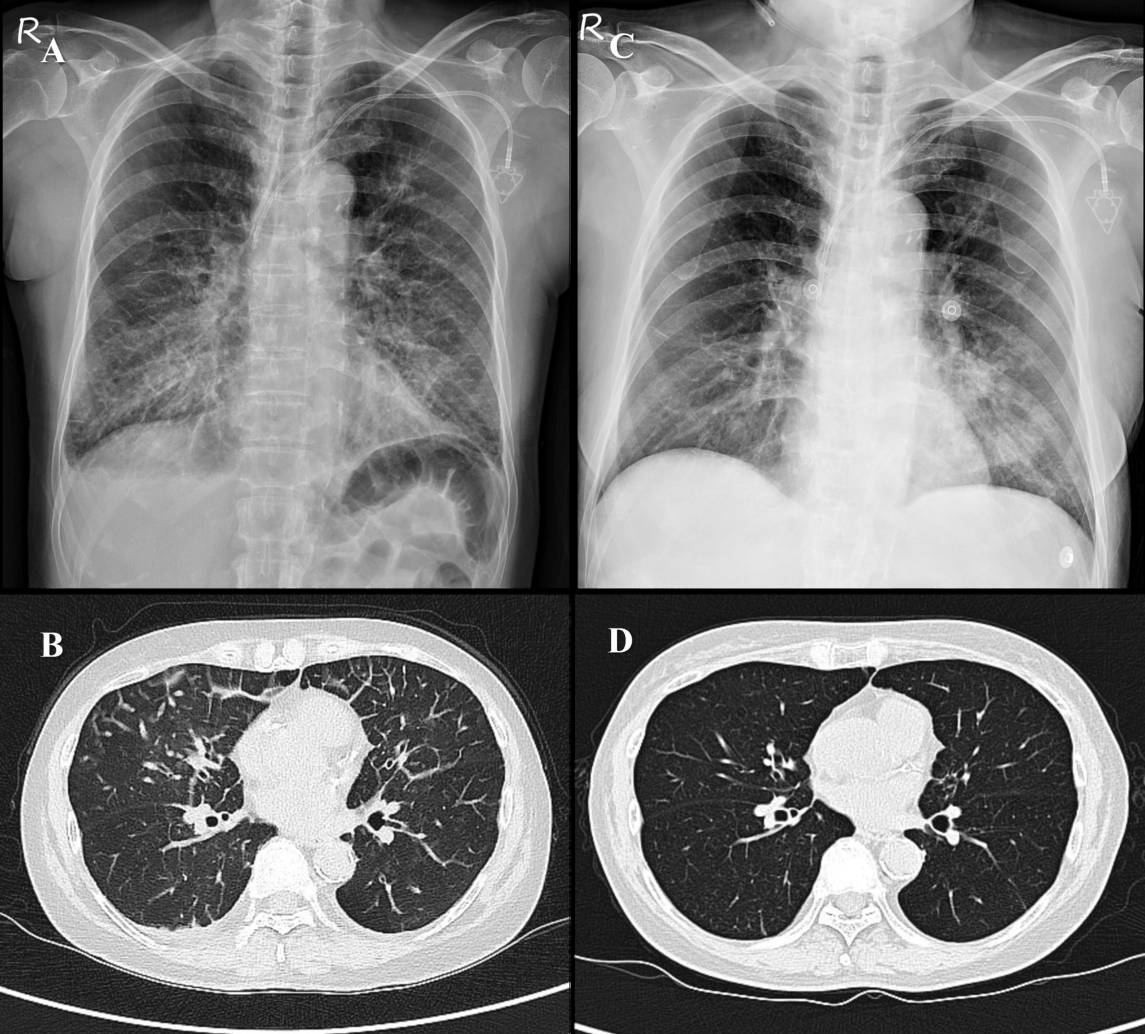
Radiographic findings before and after IVIG and high‐dose corticosteroid treatment. (A) Initial chest x‐ray showed diffuse lung opacities. (B) Baseline CT scan revealed bilateral interlobular septal thickening and ground‐glass opacities in both lungs. (C) Chest x‐ray on day 10 post‐treatment demonstrating significant resolution of opacities. (D) Follow‐up CT at 3 months revealed complete resolution of pulmonary infiltrates.
